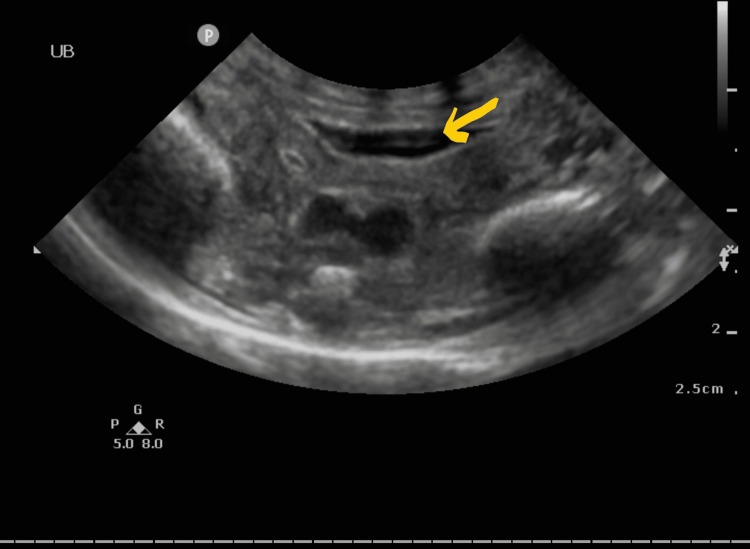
Ultrasound showing almost empty bladder despite the baby being anuric
